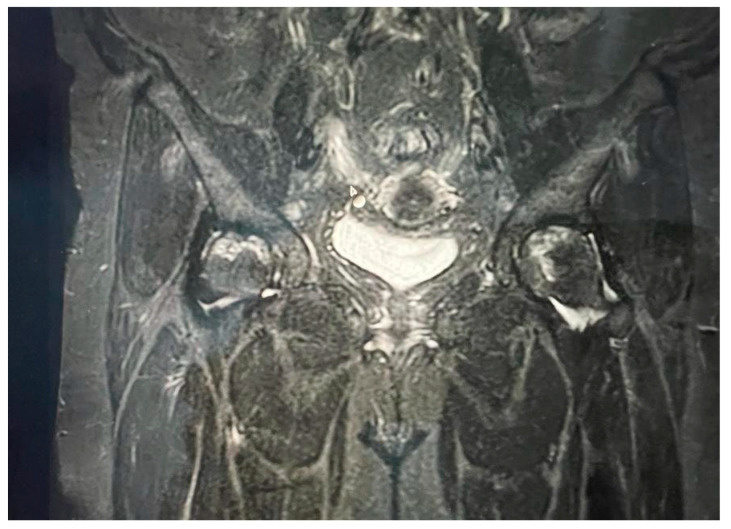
Bilateral hip MRI with avascular necrosis.
